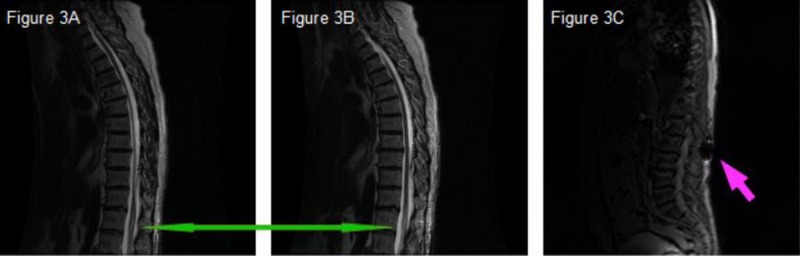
Syrinx and needle insertion during the explant/revision (A-B) Green arrow directed to syrinx on sagittal T2-weight MRI images. (C) Gradient echo sequence with pink arrow directed at needle insertion point at L2/L3 spinal interspace directed rostrally.
